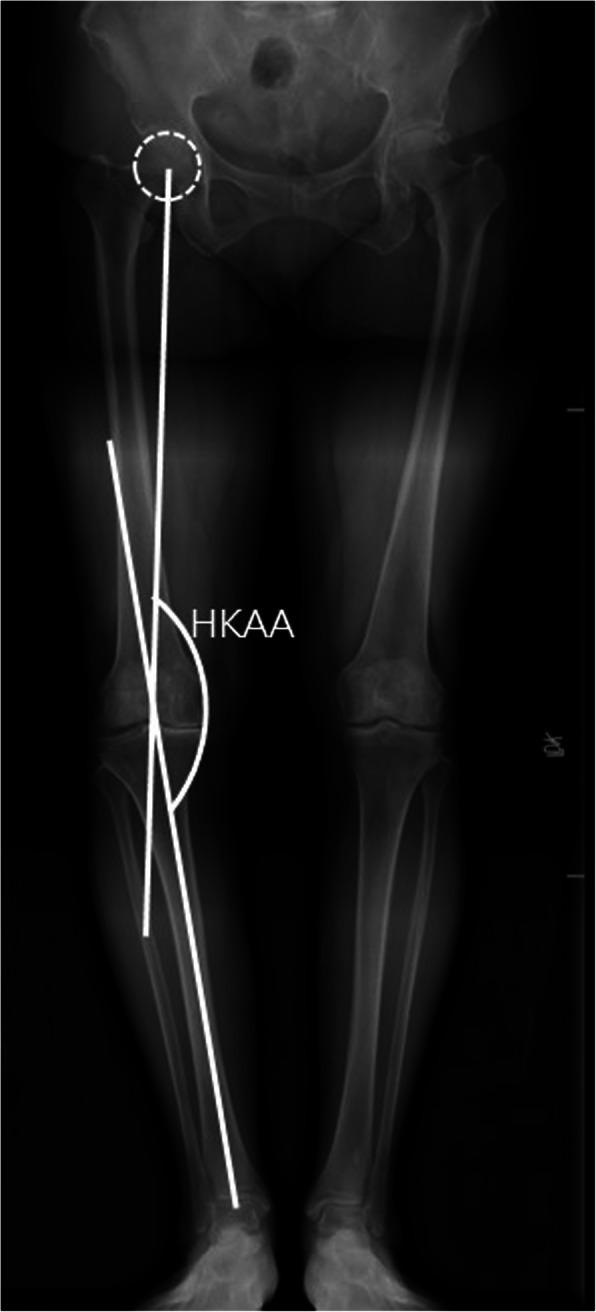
The hip-knee-ankle angle (HKAA) is measured on the whole-leg weight-bearing radiograph. It is defined as the medial angle between the mechanical axis line of the femur (direct line from the center of femoral head to the top of the femoral notch) and the mechanical axis line of the tibia (line from the ankle center to the center of the tibial spines)
